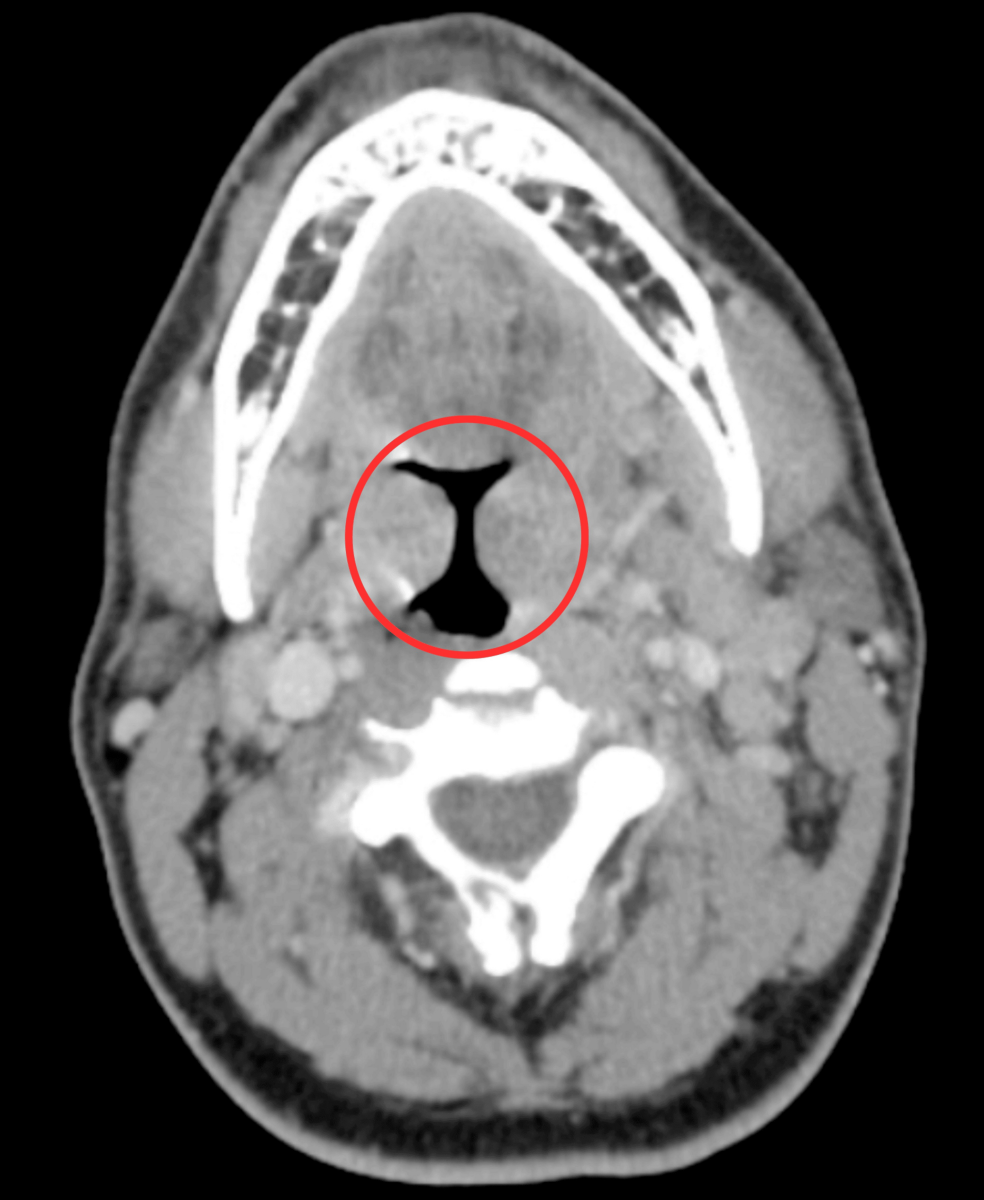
CT scan showing enlarged and heterogeneous bilateral palatine tonsils (indicated by the red circle), suggestive of acute tonsillitis CT: computed tomography
